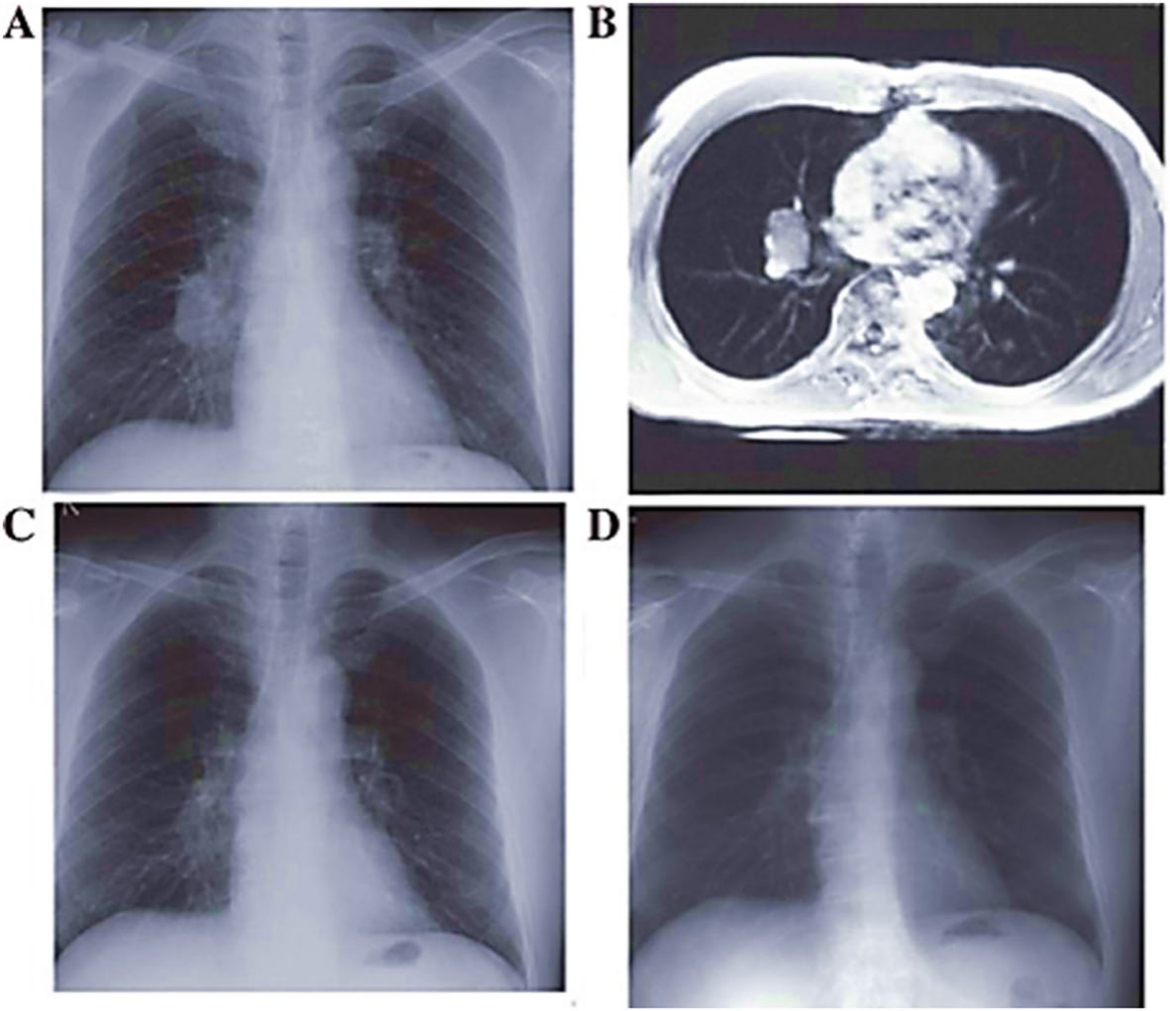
Chest X-rays and MRI (A) A lesion in the right hilus pulmonis with a clear edge is seen on a chest X-ray. B An MRI shows a nodule in the right hilum. C A chest X-ray shows no mass but a tangled network of blood vessels (D) A normal chest X-ray [85]
